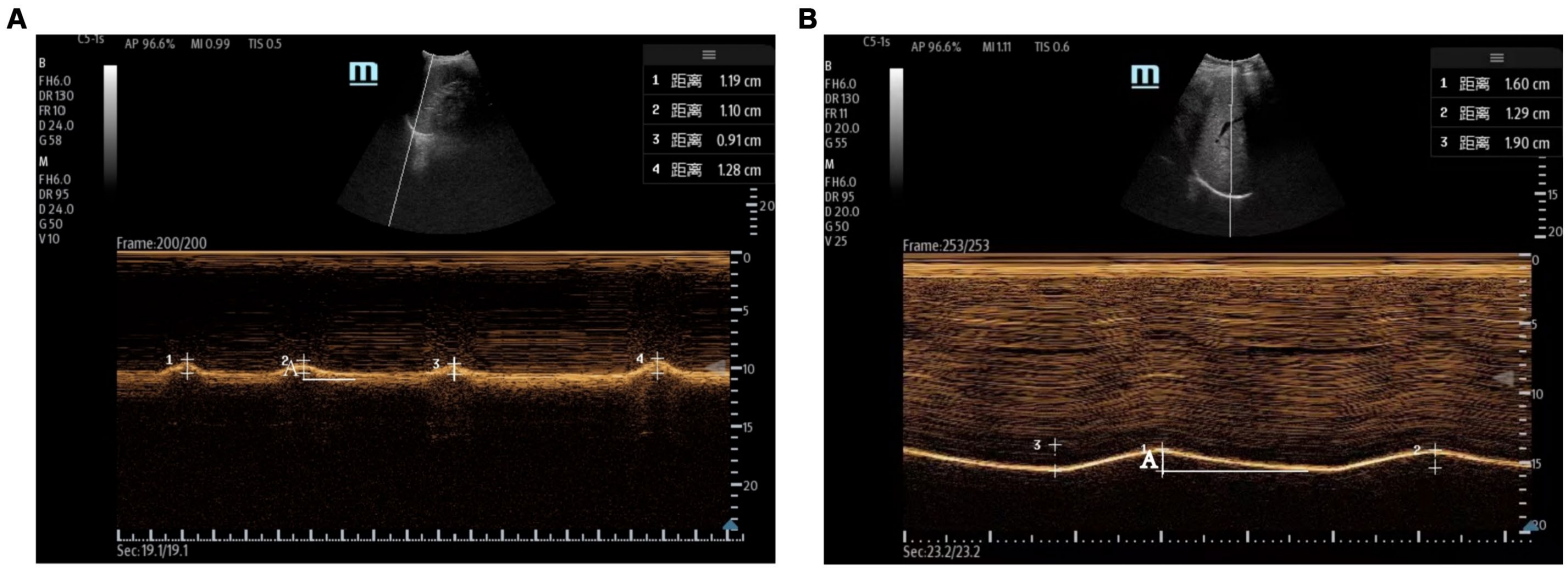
Evaluation of the diaphragm using ultrasound. (A) Left measurement of diaphragmatic excursion measured with the spleen as the acoustic window. (B) Right diaphragmatic excursion measured using the liver as an acoustic window. The total length of A is the diaphragmatic excursion for one respiratory cycle.
